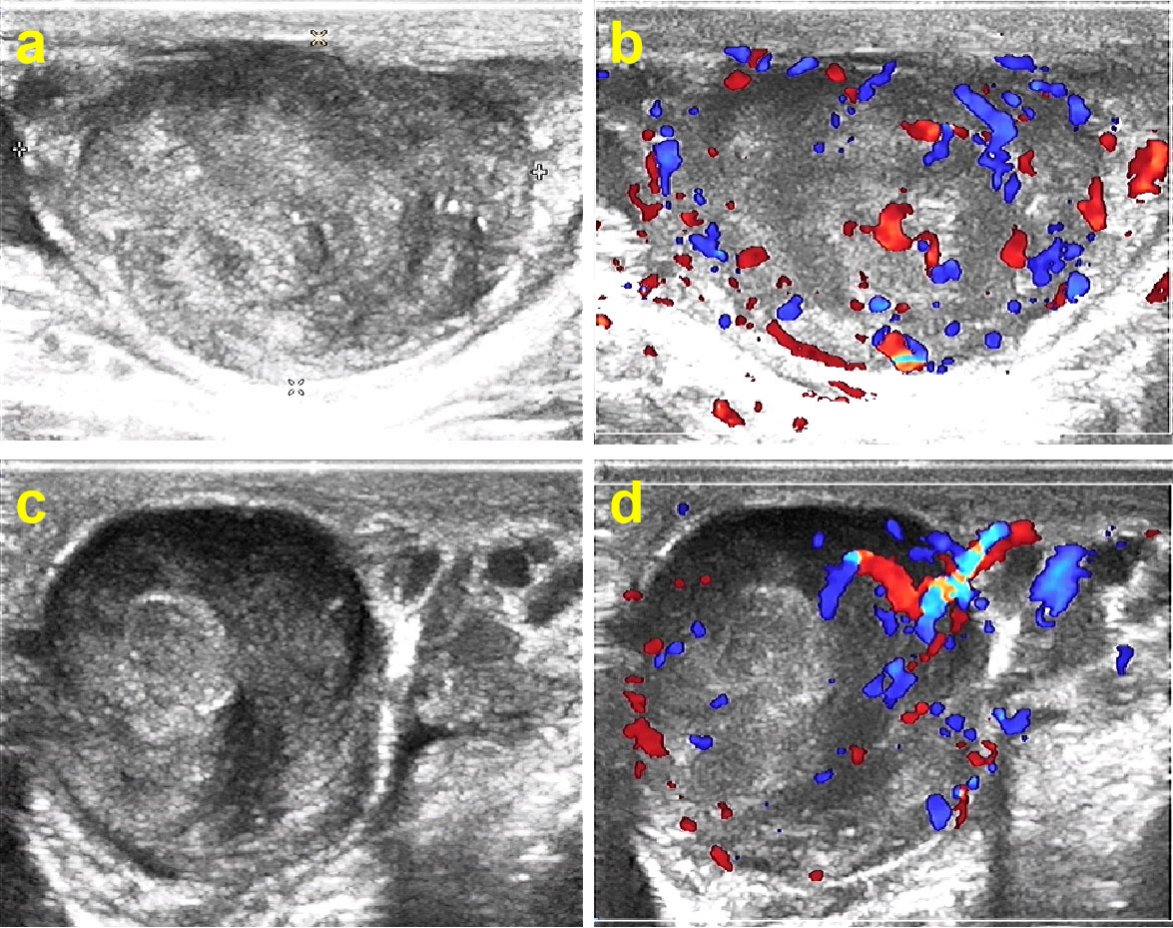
Ultrasonography of leiomyosarcoma in the caudal epididymis, (A, B) longitudinal scan, (C, D) transverse scan. There is a solid hypoechoic mass with heterogeneous internal echogenicity, clear boundaries, and abundant blood flow signals.
